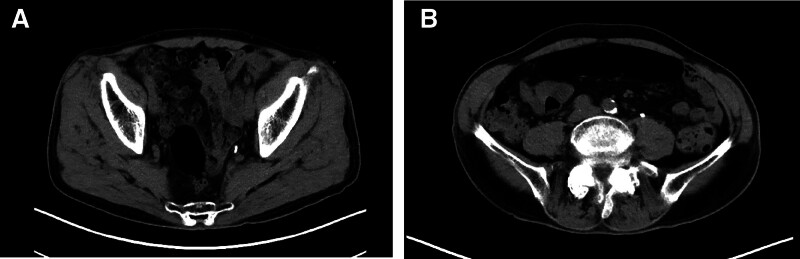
(A, B) CT scan revealed that a redundant sigmoid colon was distended. CT = computed tomography.
